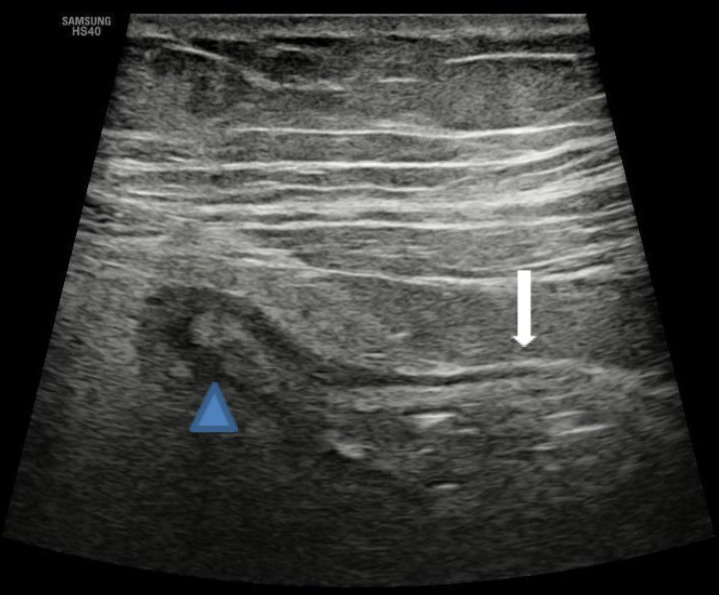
Ultrasonography of the abdomen shows a blind-ended, aperistaltic, non-compressible, tubular structure (block arrow) with surrounding inflammatory changes arising from the caecum (arrowhead).
